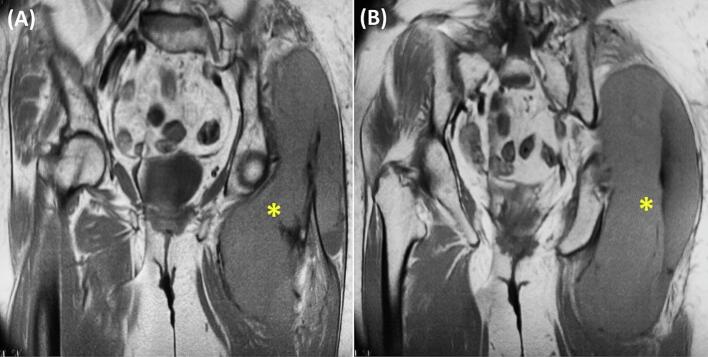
Coronal MRI findings. A: Coronal T1-weighted MRI of the pelvis showing a large soft tissue mass involving the gluteal and adductor muscles with mild hyperintensity. B: Coronal post-contrast T1-weighted MRI demonstrating heterogeneous enhancement and diffusion restriction of the mass, consistent with aggressive infiltration. Dimensions are 148 × 118 mm axially and 228 mm craniocaudally (yellow asterisk).
